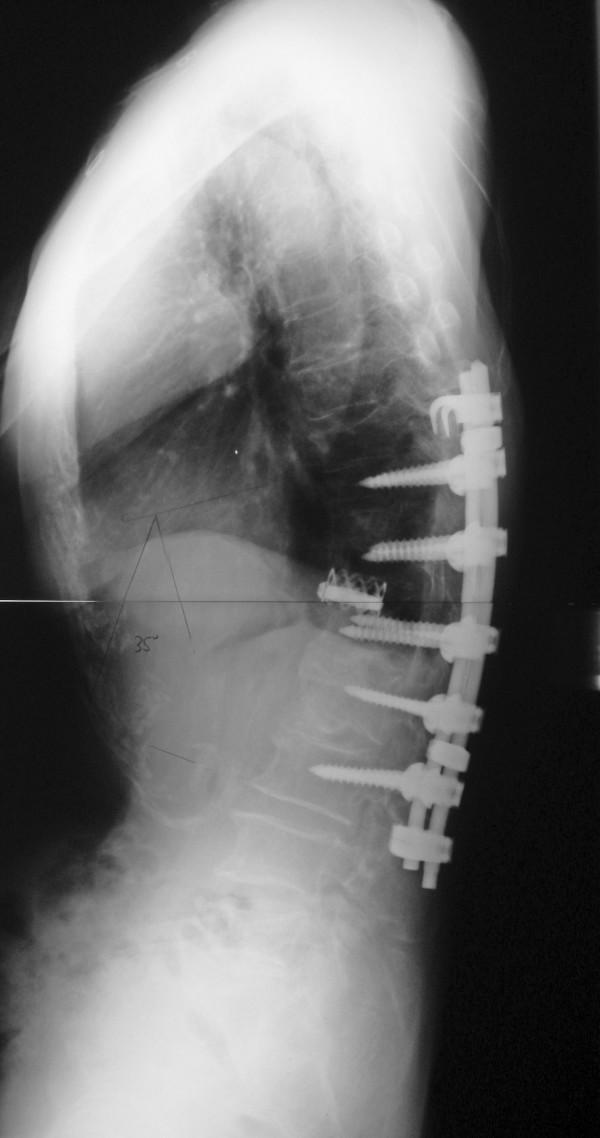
Standing lateral radiography of the spine obtained 2 years after surgery. Good correction was obtained.
